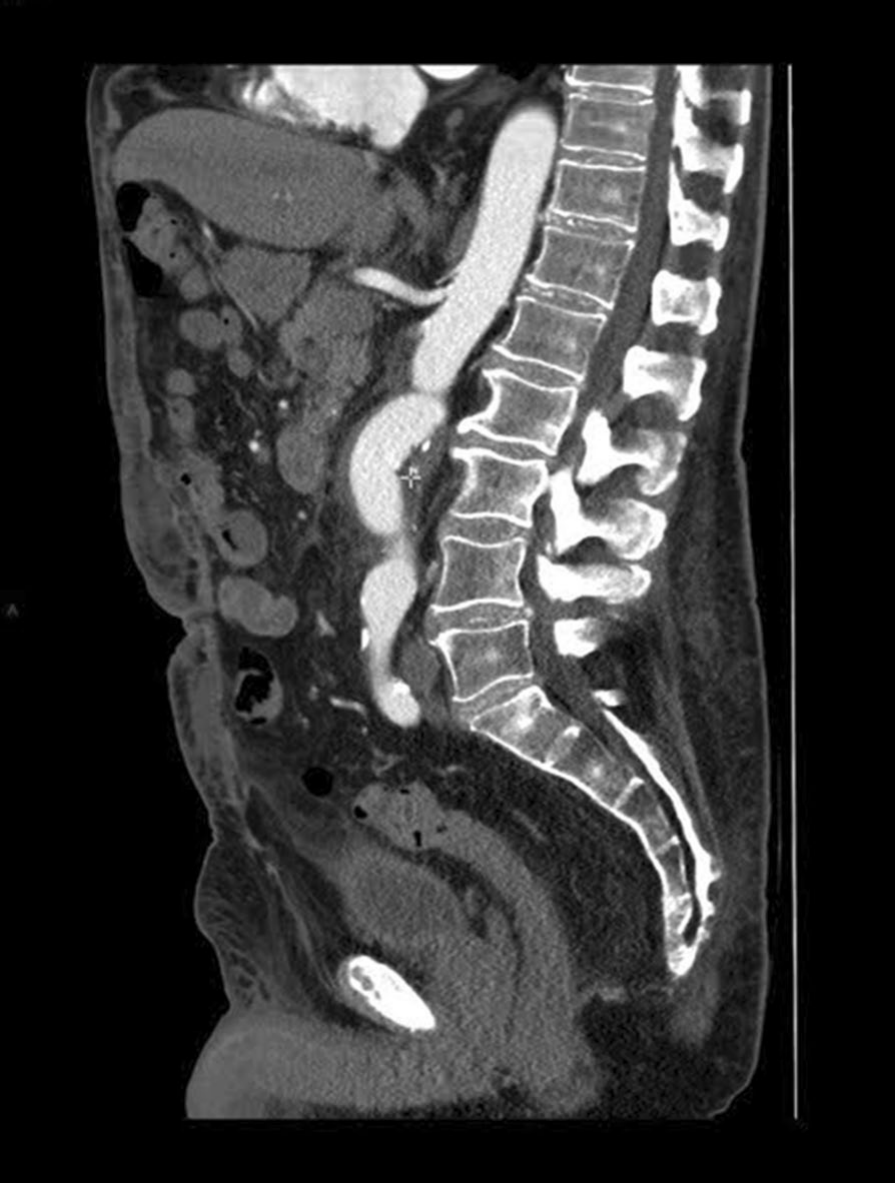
CT sagittal section performed showing abdominal aortic aneurysm. A CT was performed after initial failure of antibiotherapy and showed and abdominal aortic aneurysm previously seen on US
